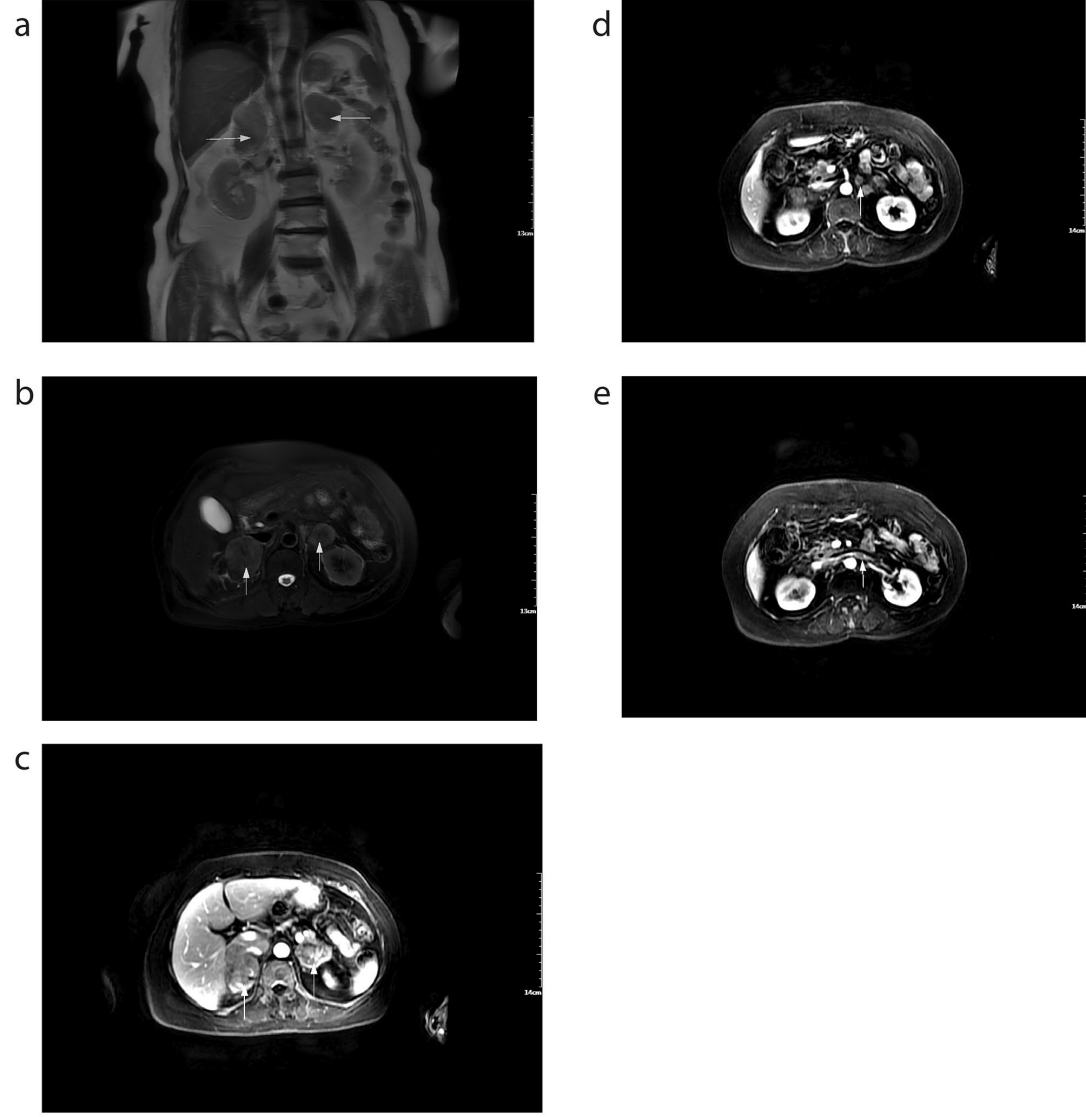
(A, B)Contrast-enhanced MR confirmed a long T1 and T2 signal shadow (arrow). Contrast-enhanced MRI revealed moderate enhancement in adrenal masses (C,arrow) and tumor thrombus in the central adrenal vein (D,arrow) and left renal vein (E,arrow)
